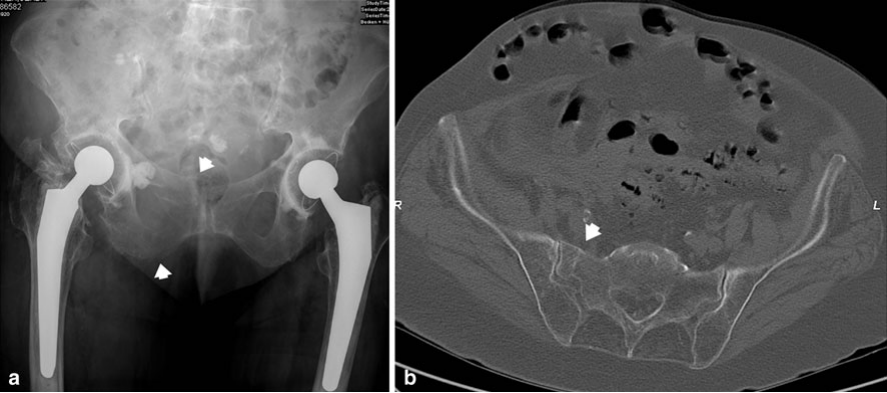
a Pelvic overview of 91-year-old female with severe osteoporosis. Pubic and ischial rami fractures with slight displacement are visible (*arrows*). b Transverse CT-cuts through the sacrum showing a vertical fracture in the sacral ala (*arrow*). The patient was treated conservatively
